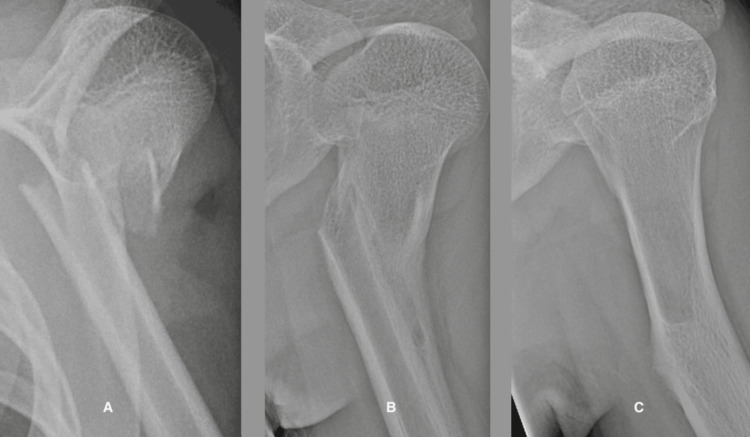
A 10-year-old girl sustained a completely displaced proximal humeral fracture. A. The initial radiograph shows a 100% displacement of the fracture. B. Five months later, she has full painless shoulder motion. C. Two years after the fracture, only a small medial cortical irregularity marks the original medial cortical spike.
